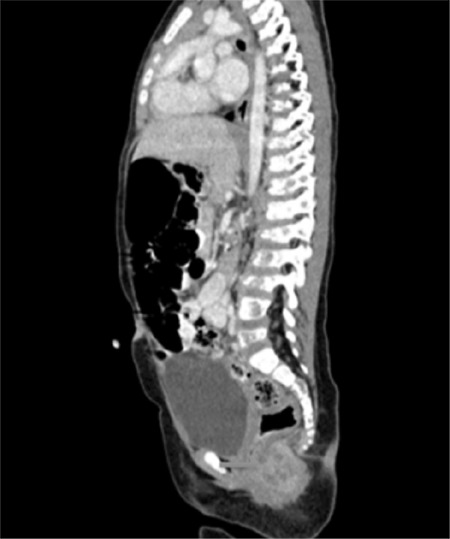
Abdominal CT showed a huge mass in pararectal fossa. CT: Computed tomography.
